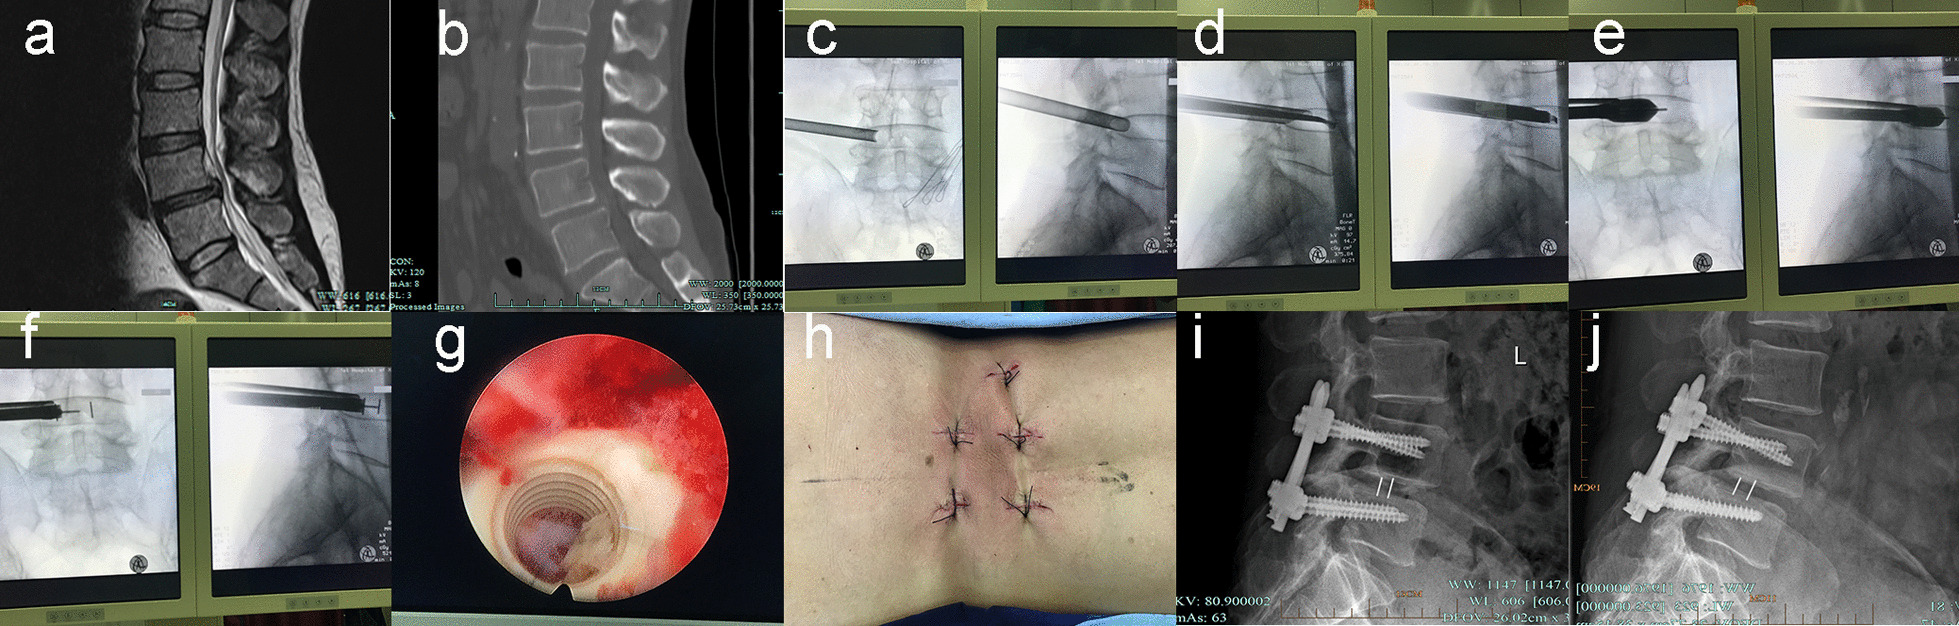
Typical case 2. A 53-year-old male with recurrent low back pain accompanied by right lower limb radiating numbness and pain for six months, aggravating for 10 days. Sagittal MRI and CT show disc herniation and instability at L4/5 level (a, b). Achieve the foraminoplasty and laminectomy to have enough room for decompression (c). Clear the disc space with a scraper (d). Test and adjust the mould of cage by intraoperative fluoroscopy (e). Implant cage with working channel or nerve root retractor (f). Perform secondary endoscopic exploration and clear the disc space (g). Postoperative surgical incision (h). Lateral X-ray one month after surgery (i). Lateral X-ray 1 year after surgery (j)
